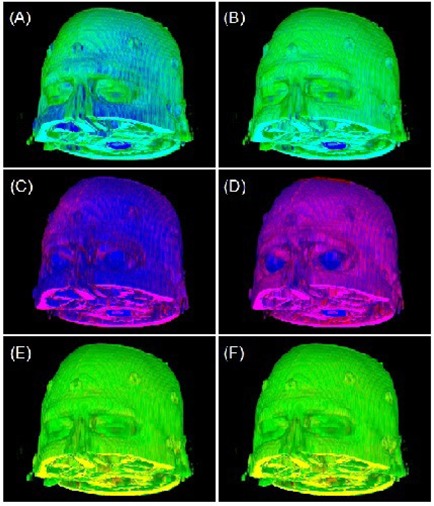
Correction of misalignments among three co‐registered MRI images: T1 (green), T2 (blue), and FLAIR (red). The original images are shown on the left column (Figs. (a), (c) and (e)) and the corrected images are shown on the right column (Figs. (b), (d) and (f)).
